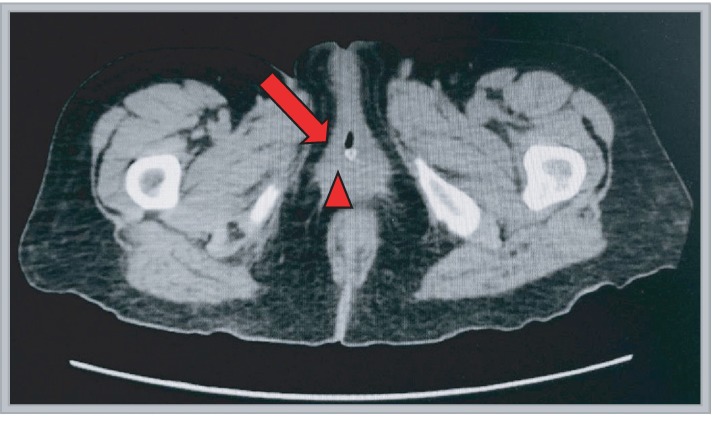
Axial cut CT image showing the urethral defect (*red arrow*) just anterior and slightly lateral to the Foley catheter (*arrowhead*) in place.
